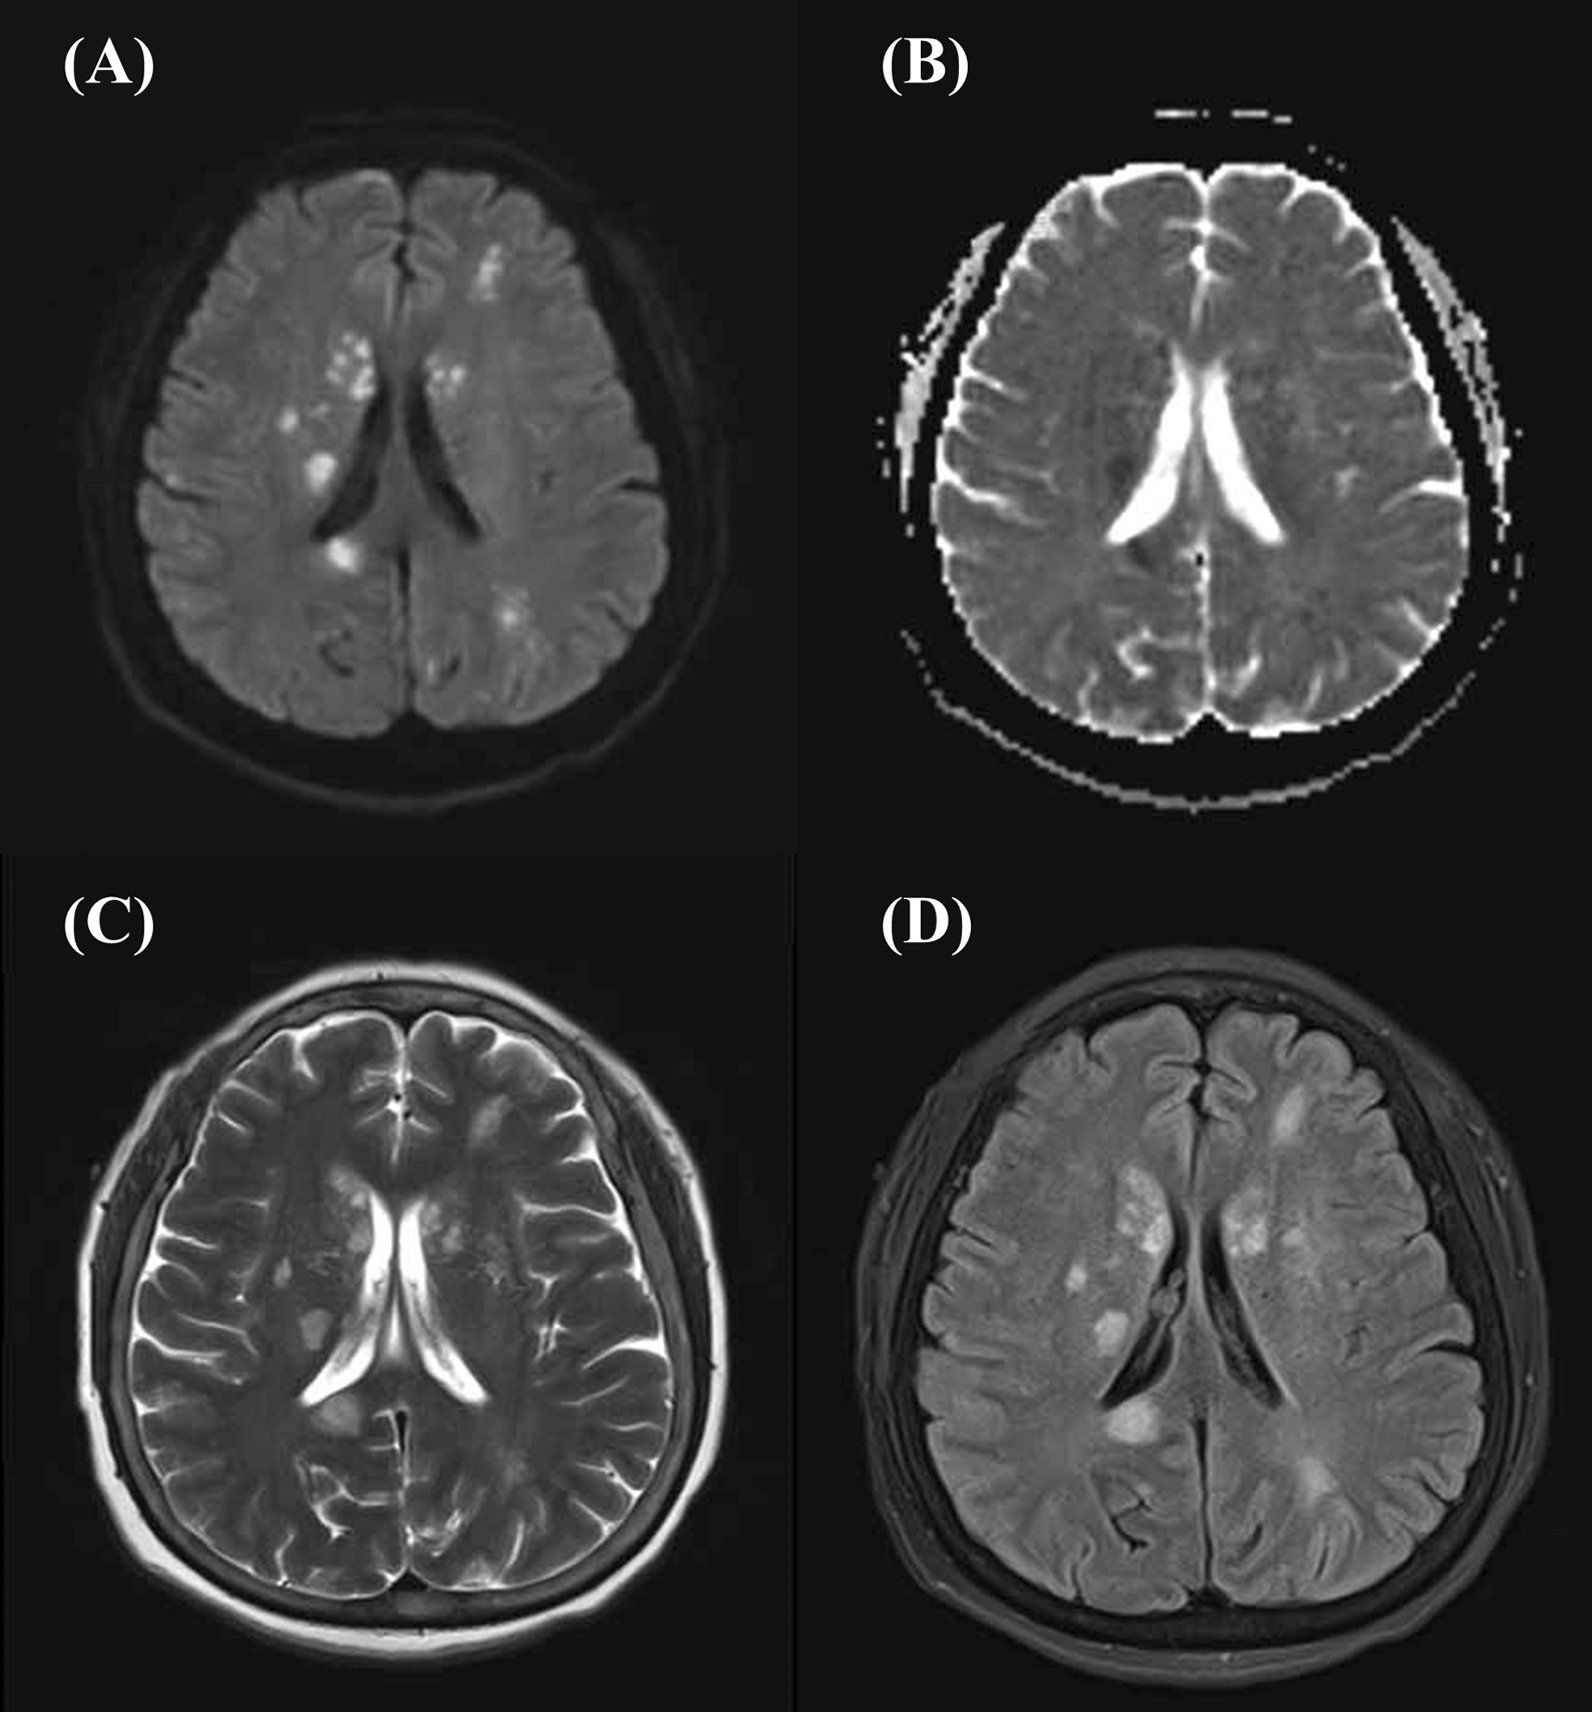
Brain magnetic resonance imaging. A Diffuse-weighted image (DWI), B apparent diffusion coefficient (ADC) map, C T2-weighted image, and D fluid attenuated inversion recovery (FLAIR) image. Multifocal lesions of high intensity on DWI and ADC maps showed low values. There were multiple T2 high signal lesions in bilateral cerebral white matter
